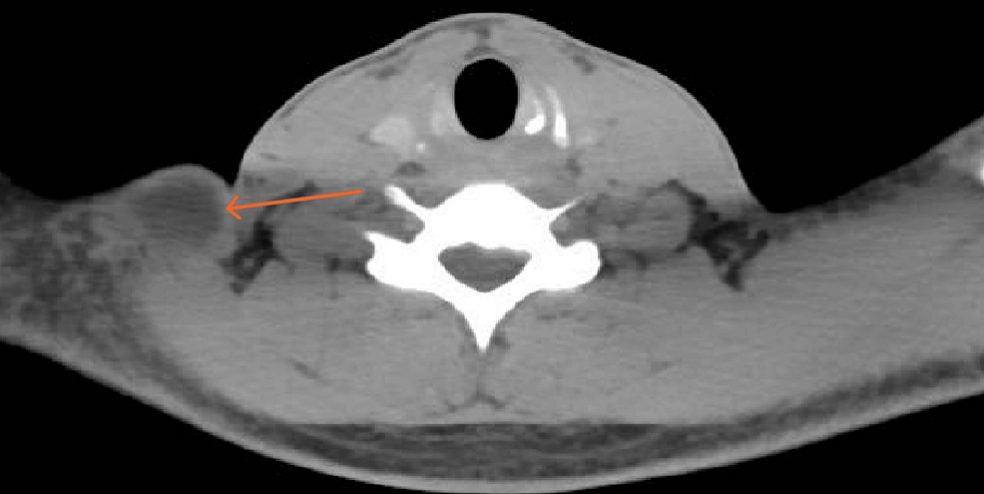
Axial cut CT of the neck demonstrates 2.5 cm × 2.4 cm cystic lesion noted in the subcutaneous right lower neck. No septation, No calcification.
